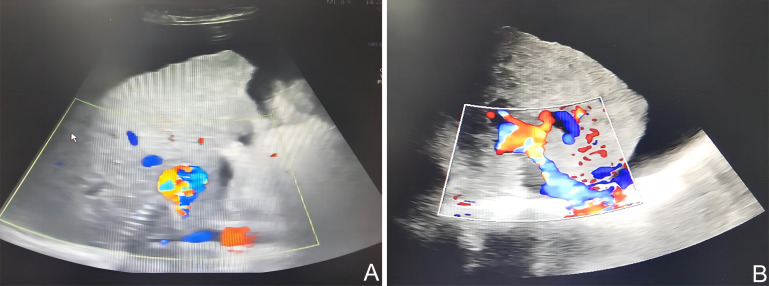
The presence of portal vein thrombosis was evaluated using color Doppler ultrasound.
